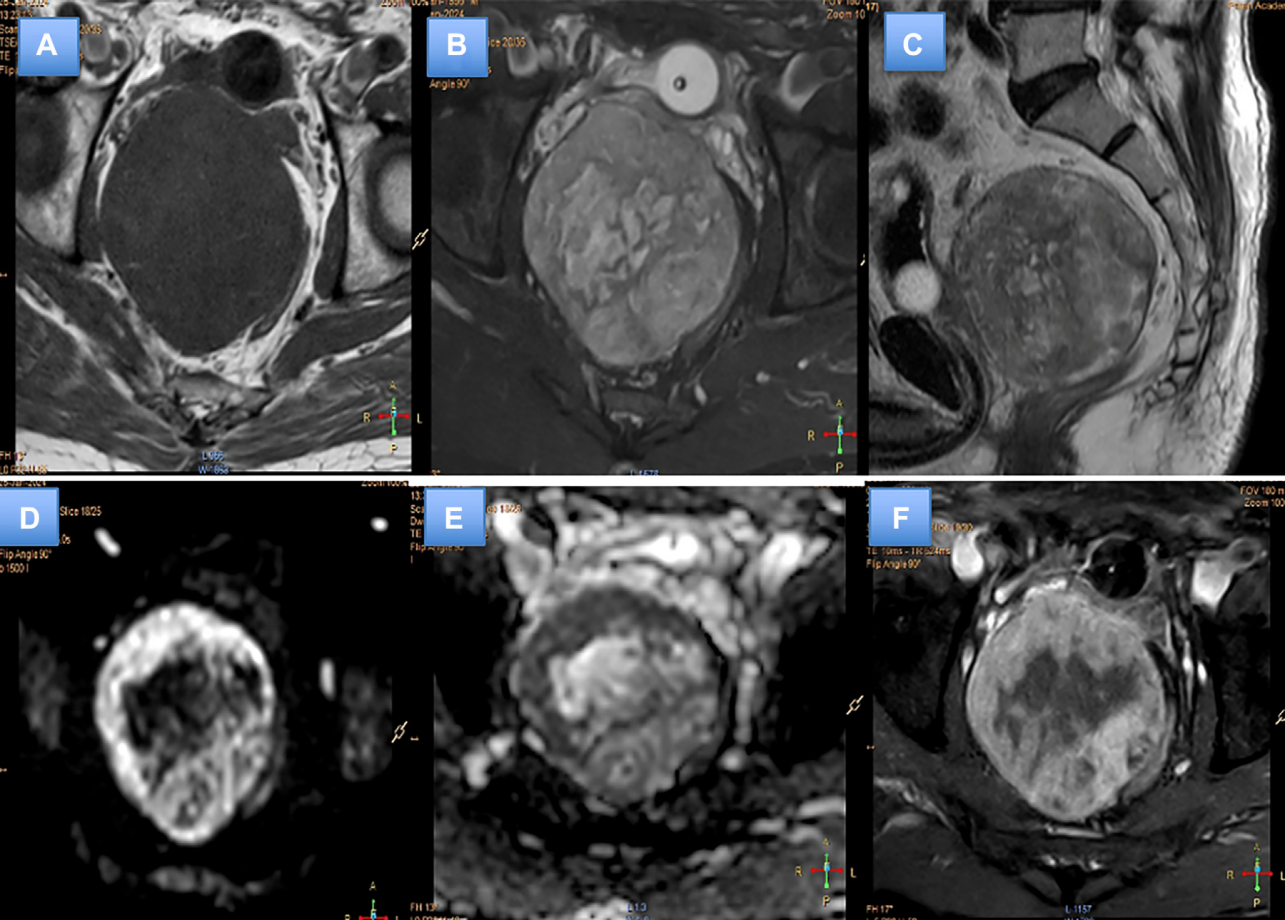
Plain and contrast enhanced MRI of pelvis showing well defined T1 hypointense (A), T2 heterogenous (B) with interspersed T2 hyperintense lesion displacing the urinary bladder and prostate anteriorly. Peripheral diffusion restriction with high signal on periphery (D) and corresponding ADC hypointensity (E) on b-value of 1000. Postcontrast study (F) showing heterogenous enhancement with central nonenhancing areas.
